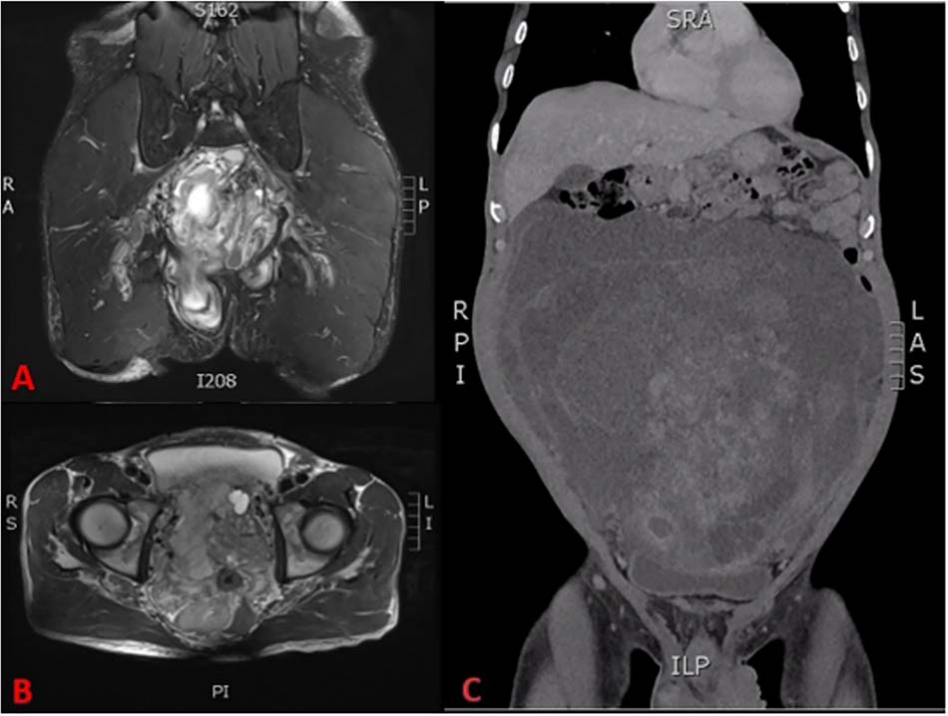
(A) T2 coronal section of MRI pelvis showing tumour invasion of the pelvic floor, (B) T2 axial MRI pelvis showing tumour involvement of the left seminal vesicle and, (C) coronal CT scan showing compression of the bladder and tumour burden.
